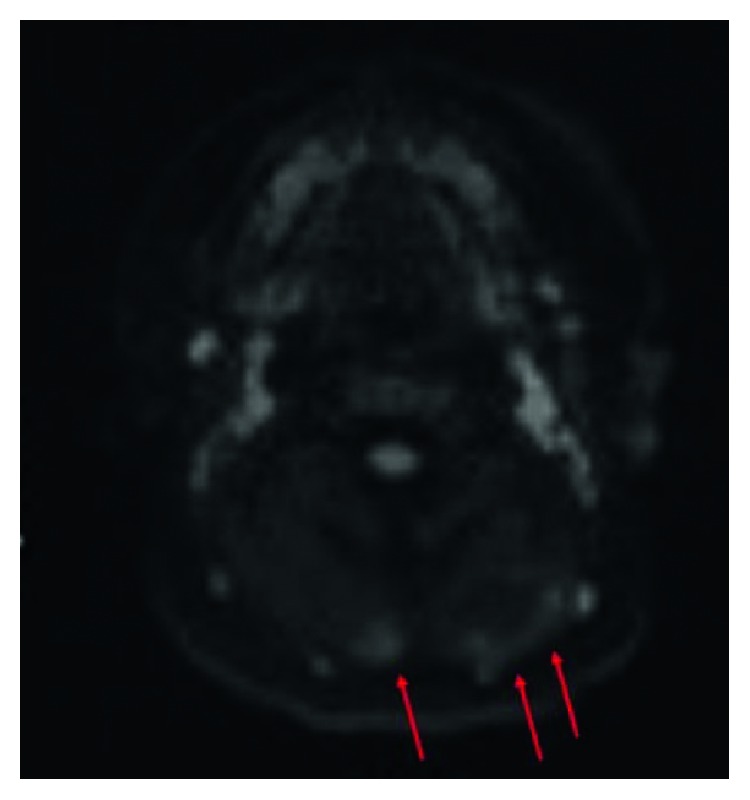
Axial diffusion-weighted imaging (DWI) demonstrates areas of rounded restricted diffusion in the posterior left and right paraspinal muscles of the neck (arrows).
